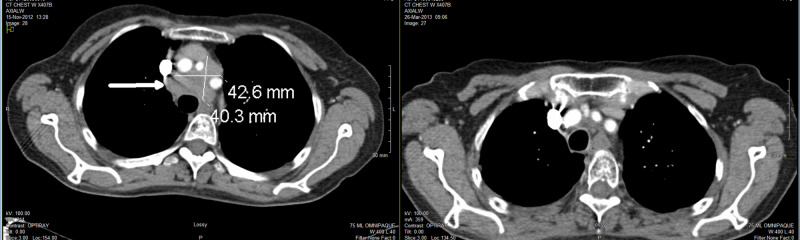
CT in November 2012 showing recurrent mass in mediastinum (image on left) and in March 2013 showing CR after palliative CTRT 30 Gy (image on right) CR, complete response; CTRT, consolidative thoracic radiotherapy
